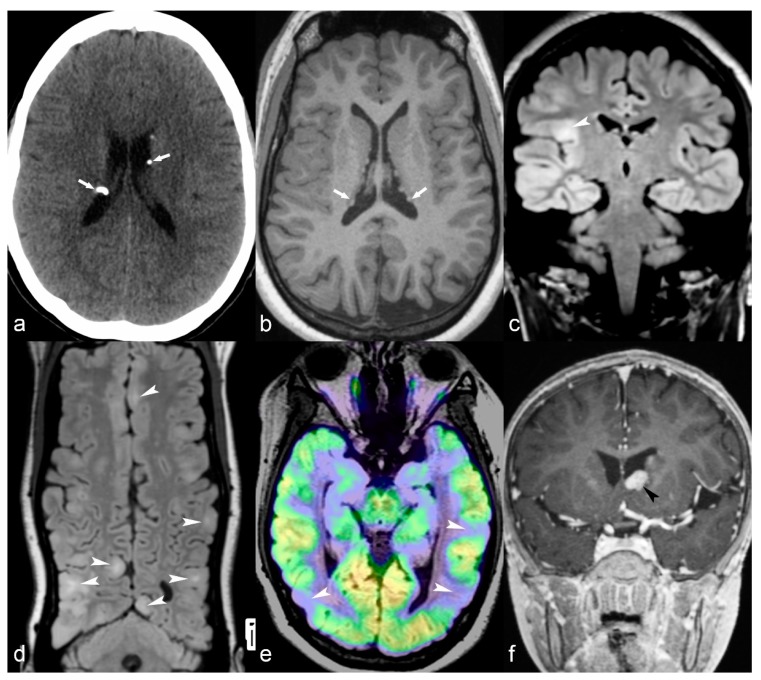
(a) 25-year-old female with Tuberosis Sclerosis Complex (TSC). Axial CT image (a) shows multiple calcified subependymal nodules (arrows). (b–e) Another 23-year-old female with TSC. Axial T1 weighted image (b) showing multiple subependymal hyperintense nodules (arrows). Coronal FLAIR (c) with curvilinear multiplanar reformat (d) shows multiple cortical tubers with radiating subcortical white matter hyperintensity (arrowheads). PET CT (e) shows hypometabolic areas corresponding to cortical tubers in bilateral occipito-temporal and left temporal region (arrows). (f) 14-year-old male with TSC. Coronal reformat of 3D T1 post contrast image (black arrowhead) shows enhancing subependymal giant cell astrocytoma abutting the floor of frontal horn of left lateral ventricle.
